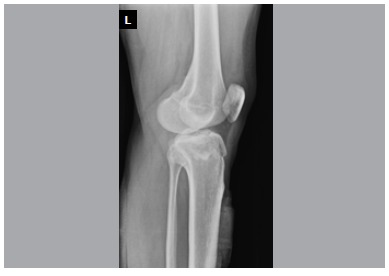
Preoperative lateral X-ray.
